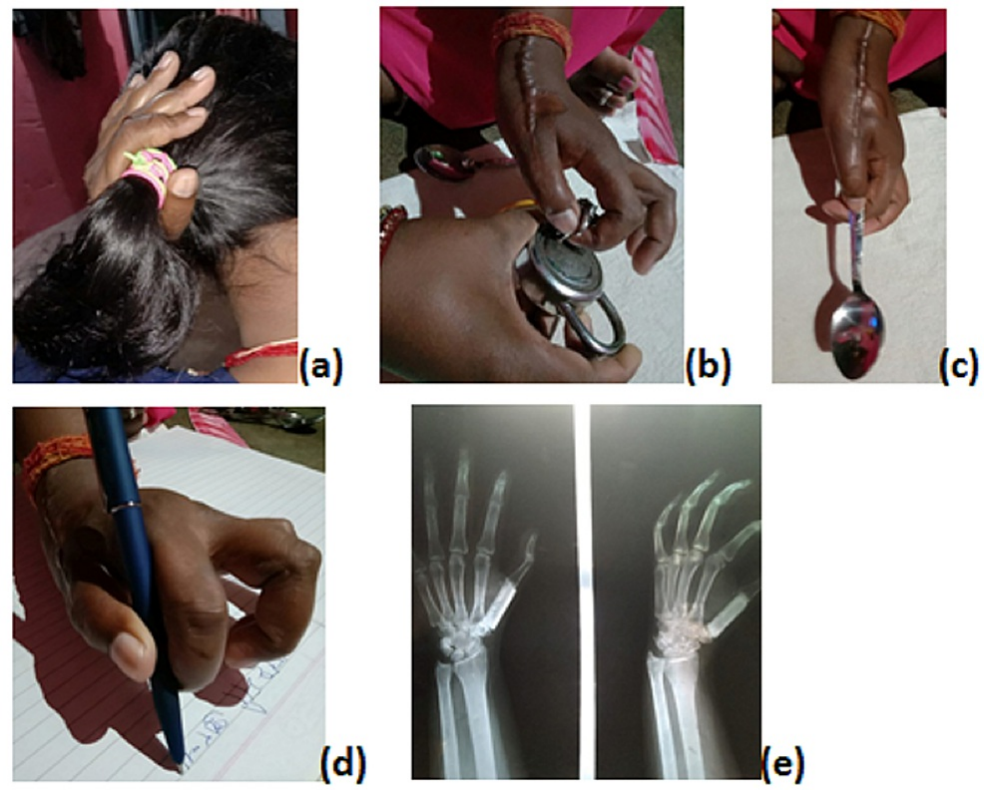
(a) One-year follow-up radiograph showing well-accepted fibula and adjacent joint fusion. (b) Functional thumb with span grip. (c,d) functional pinch grip, (e) functional ventral clenched grip.
